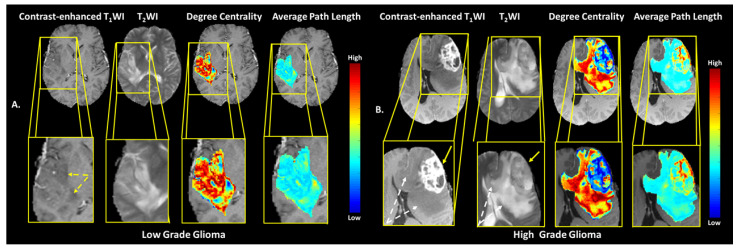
Examples of the brain MRI from the BRATS validation data set. (A) A right insular and superior temporal lobe low grade oligodendroglioma (yellow arrows) and the corresponding degree centrality and average path length maps overlaid on postcontrast T_1_ -weighted anatomic images. (B) A WHO Grade 4 left frontal lobe glioblastoma with the TCF maps of the metrics degree centrality and average path length overlaid on the postcontrast T_1_-weighted anatomic images displaying the tumor. The nonenhancing T_2_ -hyperintense peritumoral edema is shown by the dotted white arrows in the Grade 4 lesion.
